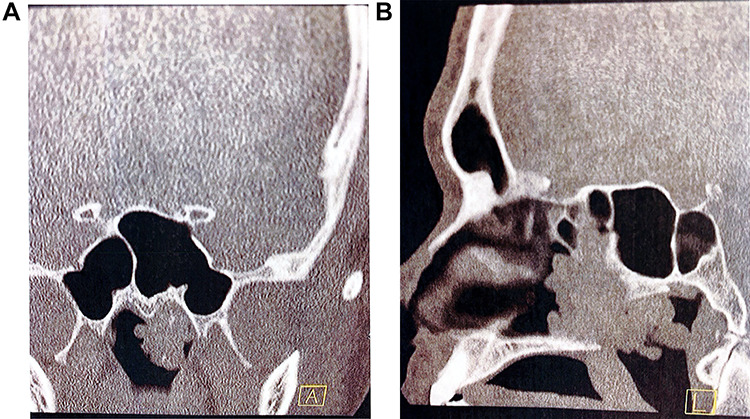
Pre-operative computed tomography of the nasal passage and sinuses. (A) Coronal CT sinuses (bone algorithm) demonstrating left nasopharyngeal space ‘polypoid’ type opacification, with evidence of intralesional calcification; (B) sagittal CT sinuses (bone algorithm) demonstrating a large polypoidal type opacification obstructing the posterior nasal choanae.
